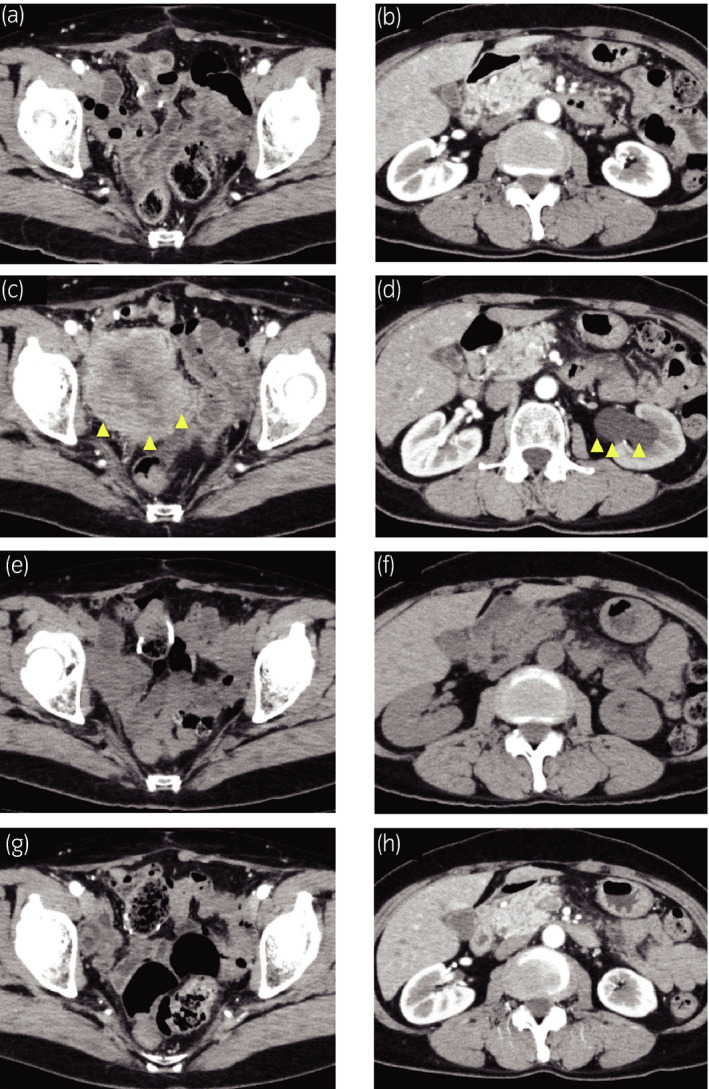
Radiological findings after LRC. (a) Abdominal CT showed no recurrent tumor 4 months after LRC. (b) No evidence of left hydronephrosis 4 months after LRC. (c) Abdominal CT with contrast showed a 60‐mm mass at the pelvis and (d) left hydronephrosis 7.5 months after LRC. (e) Abdominal CT showed disappearance of recurrent tumor at the pelvis and (f) left hydronephrosis after two cycles of pembrolizumab therapy. (g, h) Abdominal CT with contrast showed no tumor recurrence after five cycles of pembrolizumab therapy.
